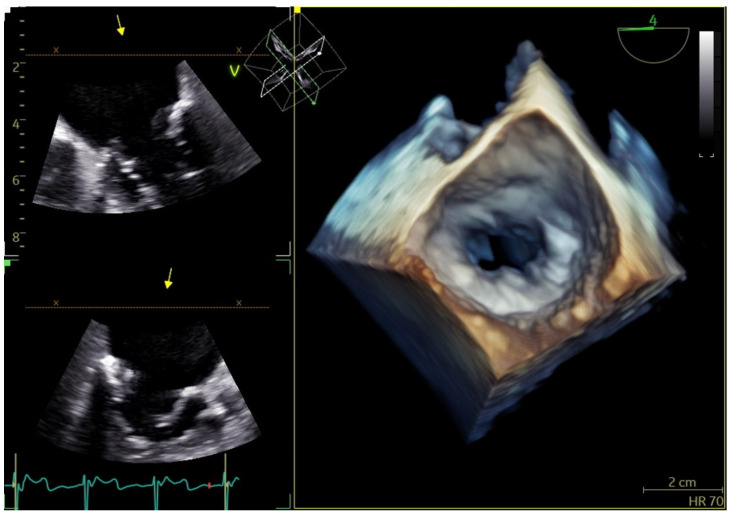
Three-dimensional TEE: severe MAC involves >270° of the annulus and results in MS due to displacement of the annulus inward. (TEE: transesophageal echocardiography, MAC: mitral annular calcification, MS: mitral stenosis).
